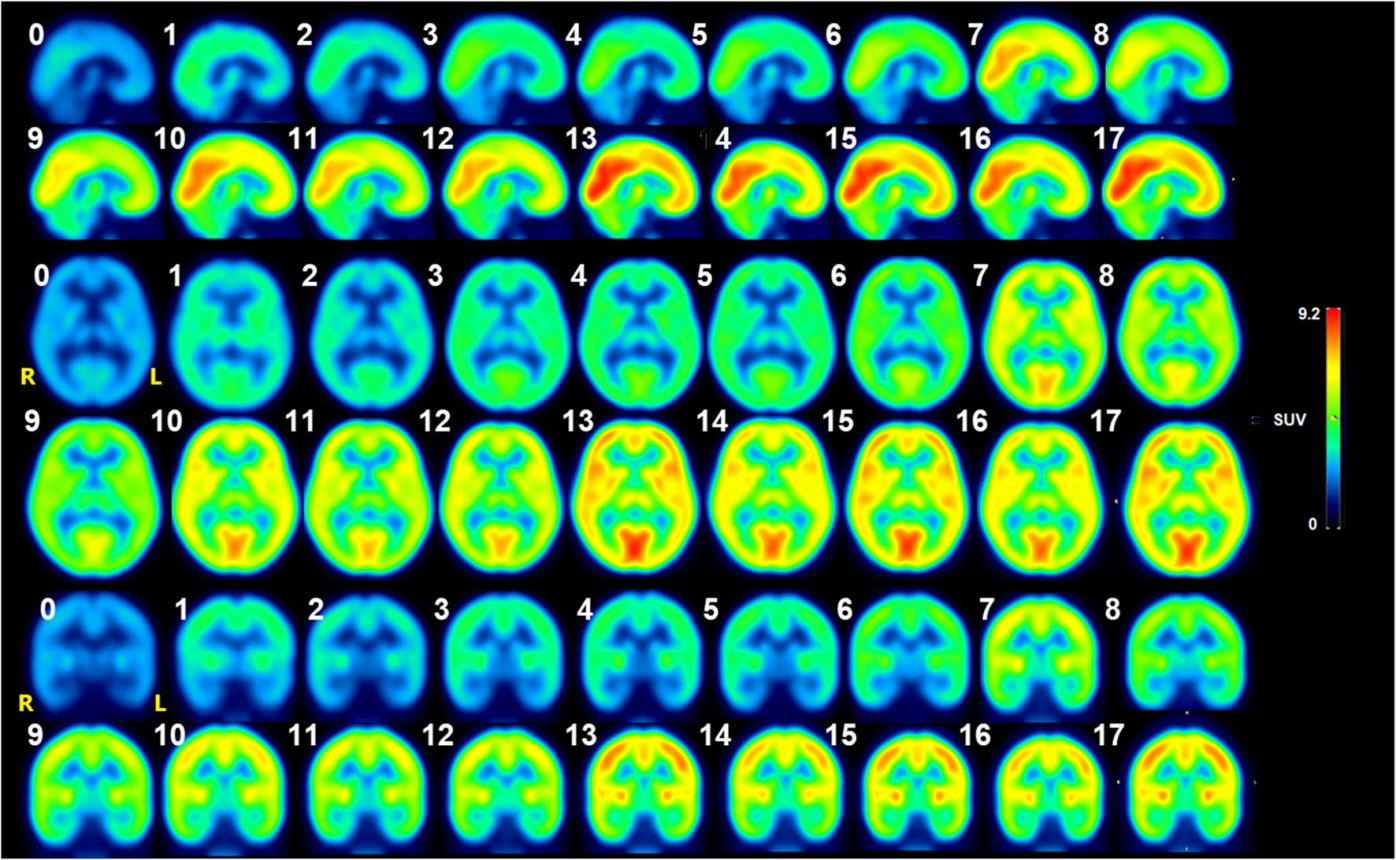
Average [^18^F]FDG-PET brain uptake image (in terms of SUV) displayed as sagittal, transverse, and coronal brain sections. Numbers indicate years of age
